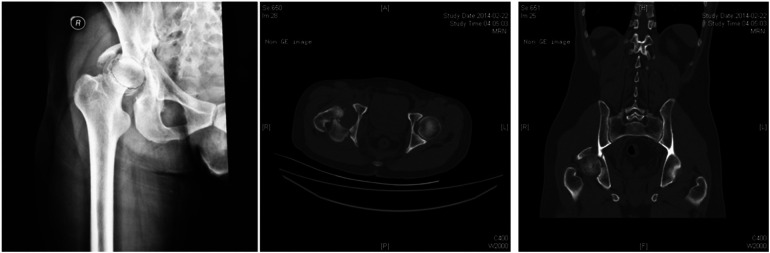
The typical case of the hip fracture triad. A 27-year-old male patient was admitted to hospital with right hip pain and limited mobility for 7 h after being injured in a car accident. He was diagnosed with right acetabular fracture, right hip dislocation, and right femoral neck fracture. Manual reduction under anesthesia was attempted in an emergency, but the reduction failed and right lower limb traction (femoral condylar traction) was used instead. Preoperative CT scan showed that acetabular fractures were classified as acetabular transverse and posterior wall fractures according to Letournel Judet classification of acetabular fractures, hip dislocation was classified as posterior hip dislocation according to the direction of femoral head dislocation, and femoral neck fractures were classified as subcephalic femoral neck fractures according to the location of the fracture line.
